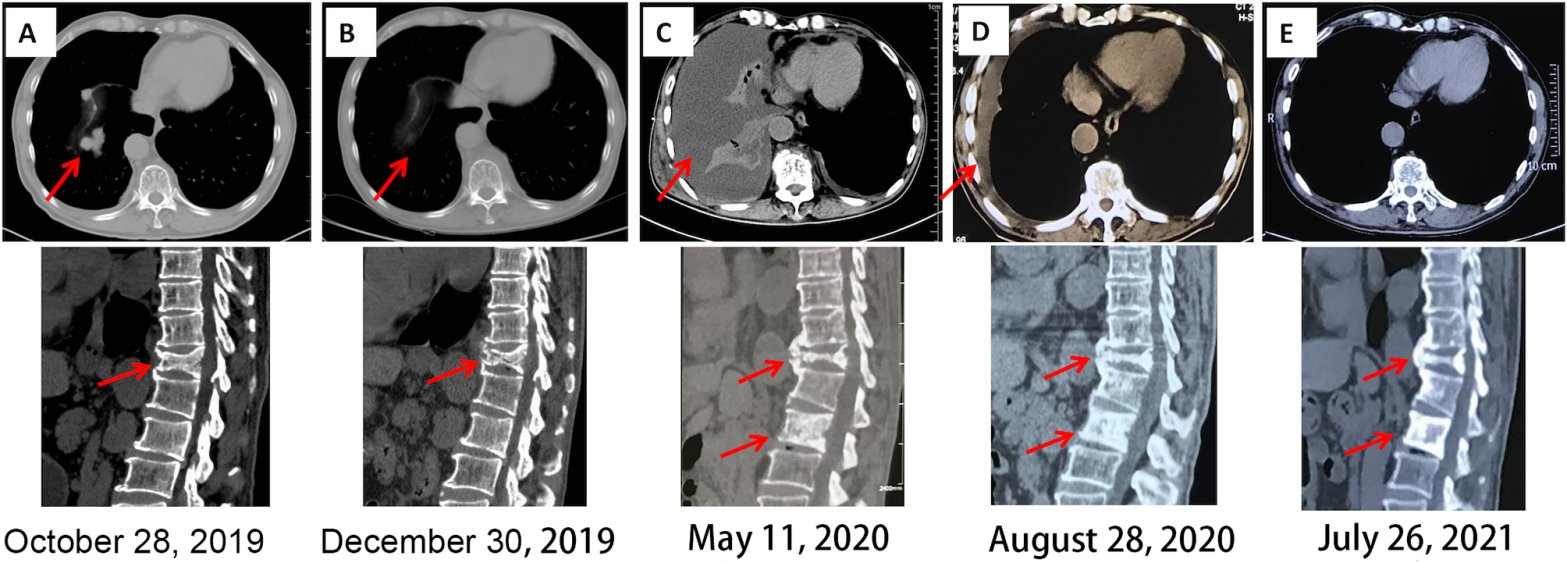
Chest and spinal CT imaging manifestations after treatment. (A) First diagnosis. (B) After one cycle of pazopanib and camrelizumab. (C) Discontinuation of camrelizumab and monotherapy involving pazopanib for 5 months. (D) Three months after the combination treatment of axitinib and sintilimab. (E) Fourteen months after the combination treatment of axitinib and sintilimab. CT, computed tomography.
